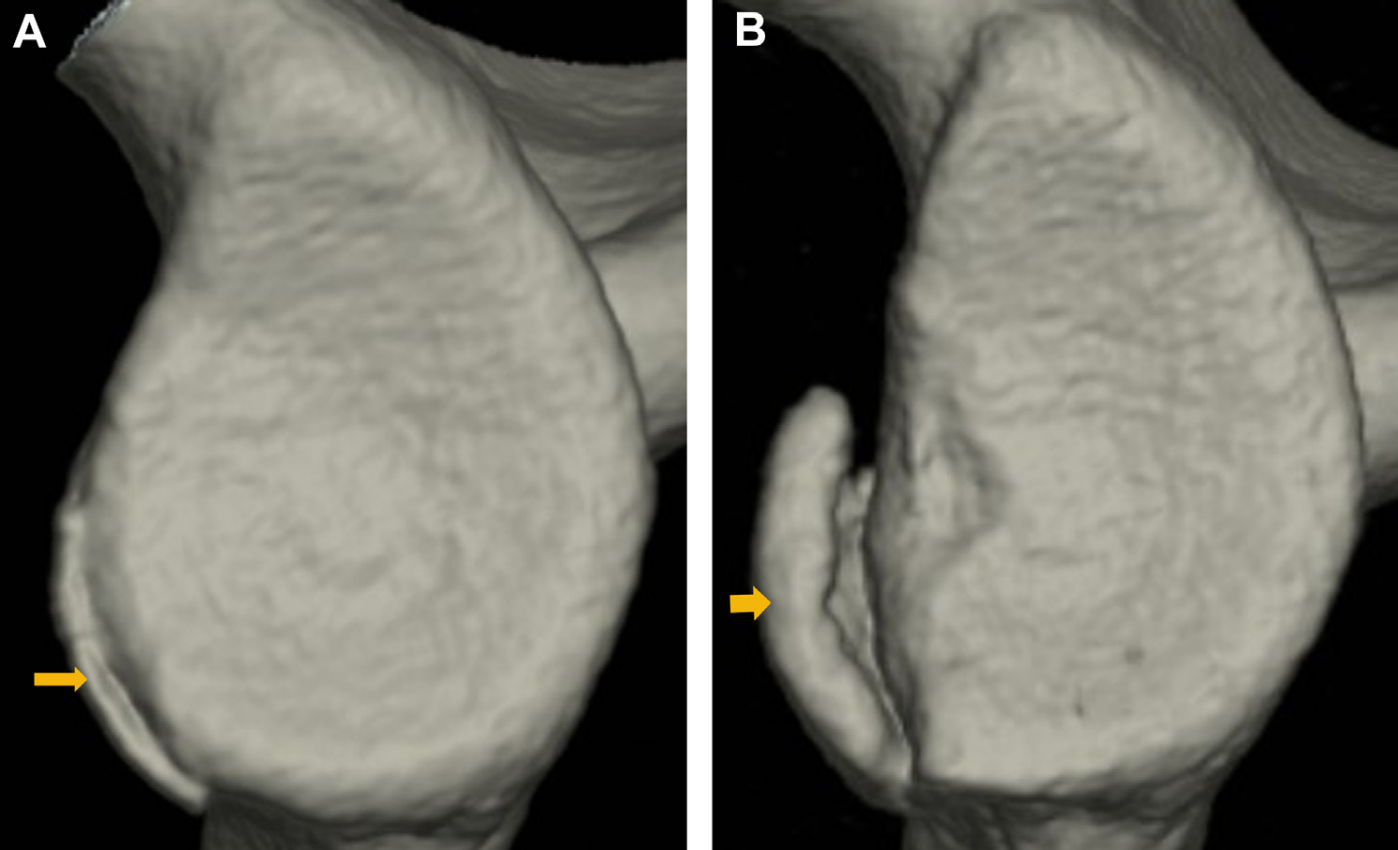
A male rugby player with an enlarged glenoid defect and enlarged bone fragment (left shoulder). (A): First CT at 1 month after primary instability (17 yr old, once instability event). (B): Second CT at 11 months after primary instability (18 yr old, total 10 instability events). At the first CT, a thin bone fragment was recognized. At the second CT, both a glenoid defect and bone fragment increased in size, while the possibility of a new glenoid fracture occurred after complete union of a primary bone fragment could not be denied. (: bone fragment). *CT*, computed tomography.
